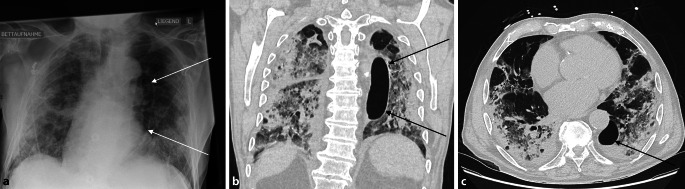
Die Obduktion ergab einen diffusen Alveolarschaden mit viropathischen Veränderungen sowie multiple subpleurale Bullae sowohl der linken Lunge als auch im rechten Lungenunterlappen. Die größte entsprach der im CT dargestellten Bulla im linken Unterlappen, welche mit 11,1 × 6,0 × 4,2 cm beschrieben wurde, ohne Hinweise auf einen Pneumothorax. Die Todesursache war das respiratorische Versagen bei SARS-CoV-2-Infektion.
